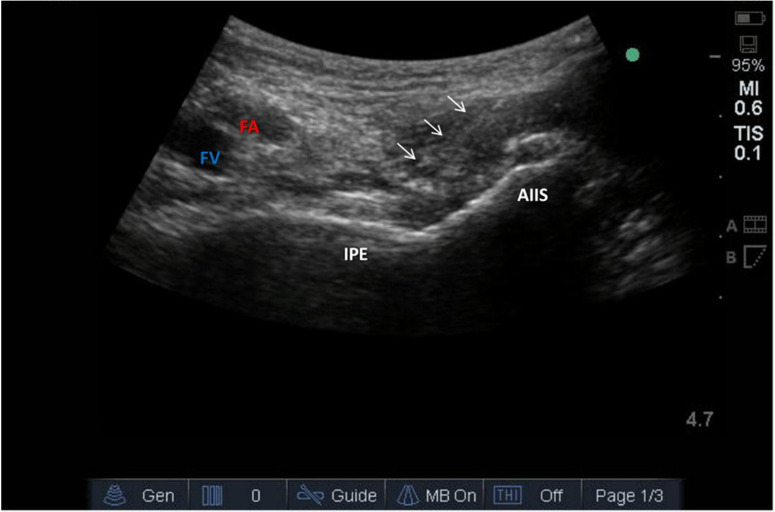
Ultrasound-guided anatomical landmarks for PENG block administration.
